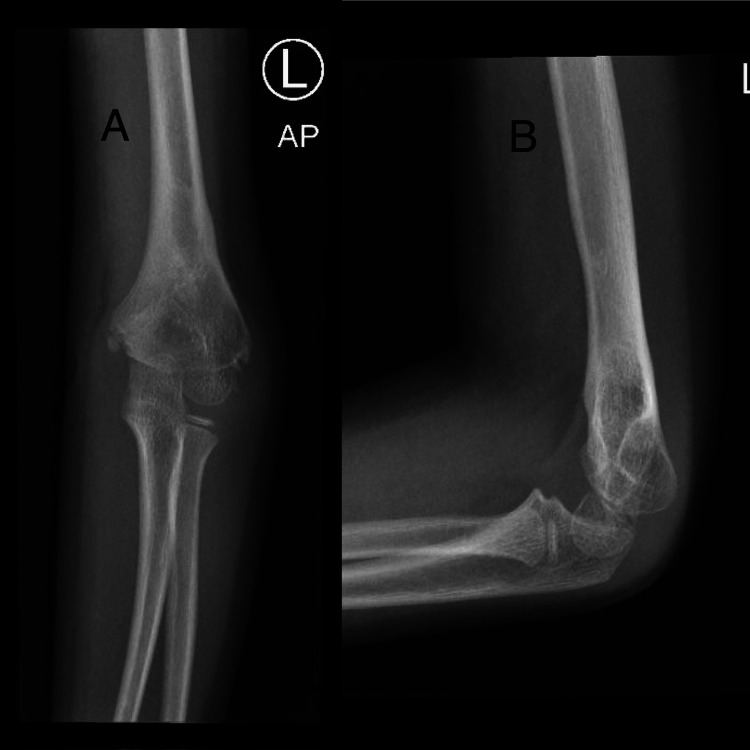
X-ray at the one-year follow-up (A) anteroposterior view; (B) lateral view
